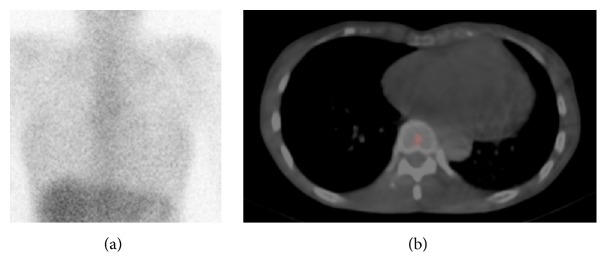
The second gallium-67 scintigraphy performed 2 months after the first scintigraphy. Gallium-67 imaging was performed 72 hours after intravenous injection with 74 MBq of gallium-67 citrate. (a) Frontal planar image of gallium-67 scan showed no abnormal accumulation of radioisotope in the heart. (b) Single photon emission computed tomography/computed tomography image of gallium-67 scan also showed no abnormal accumulation of radioisotope in the left atrial wall.
